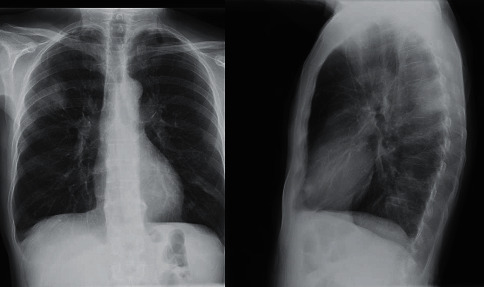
Chest X-ray showing a triangular consolidation in the right superior lobule.
